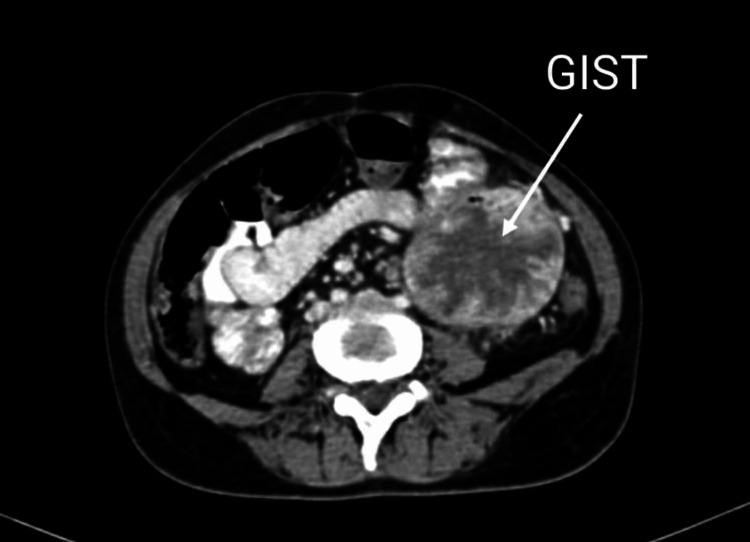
Sagittal view of the contrast-enhanced computed tomography of the abdomen showing a gastrointestinal stromal tumor (GIST) (arrow) arising from the jejunum
